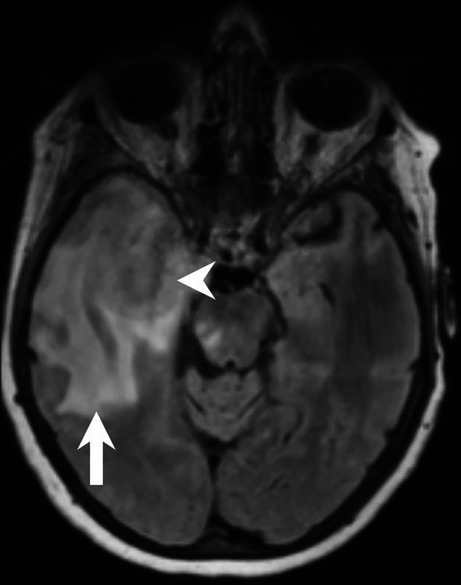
Axial fluid-attenuated inversion recovery (FLAIR) image of the head showed a large space-occupying intra-axial lesion of the right temporal lobe. The lesion (*arrowhead*) could hardly be discriminated from the extended perifocal edema (*arrow*)
